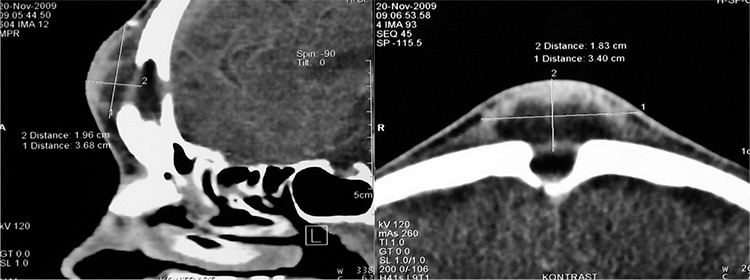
Sagittal (a) and axial (b) contrast-enhanced CT scan of the paranasal sinuses show osteomyelitis of the frontal bone with destruction of the anterior and posterior frontal sinus wall. Note the presence of fistulous communication between the subperiosteal and epidural abscess.
